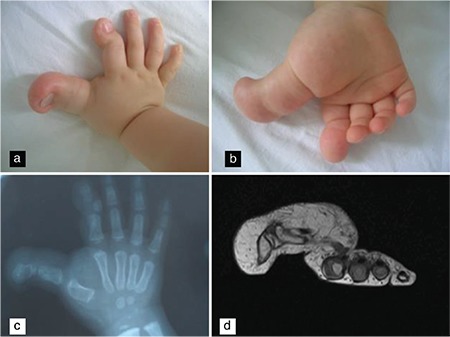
Affected fingers and thenar of the right hand showing enlargement (a, b). Radiograph of the affected fingers showing soft tissue and bony overgrowth in the metacarpal bones of the thumb and index finger (c). Magnetic resonance imaging showing accumulation of fatty tissue (d).
